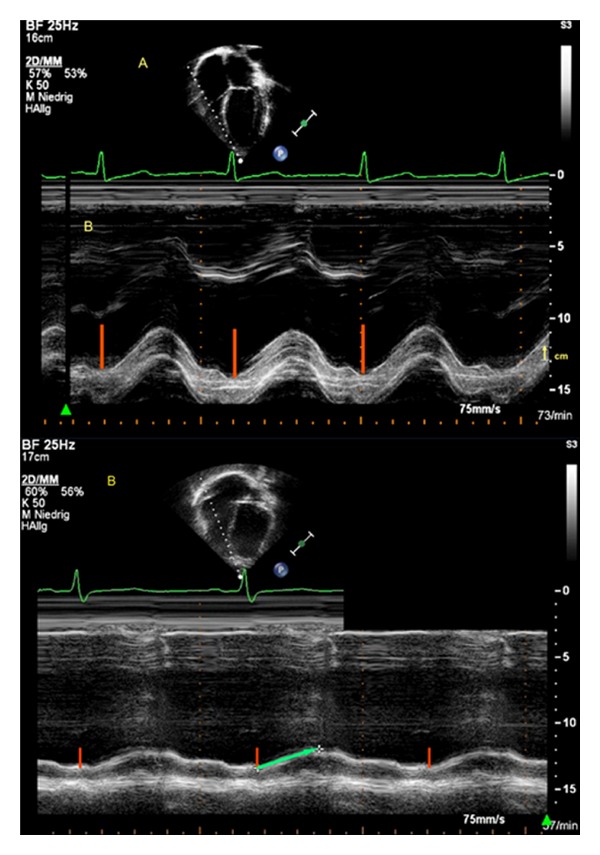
Apical 4-chamber view. (a) The white broken line indicates M-mode cursor placement at the tricuspid lateral annulus. Representative M-mode image of the tricuspid annular plane systolic excursion (TAPSE) in a patient with normal right and left ventricular function. The absolute longitudinal displacement measure is shown as the red line. The yellow arrow marks the upper and lower measure point of one centimeter (cm). (b) Representative M-mode image of the tricuspid annular plane systolic excursion (TAPSE) in a 17-year-old patient with TOF and a decreased TAPSE. The absolute longitudinal displacement measure is shown as the red line. The green arrow shows the decreased TAPSE value and flat course of the excursion.
